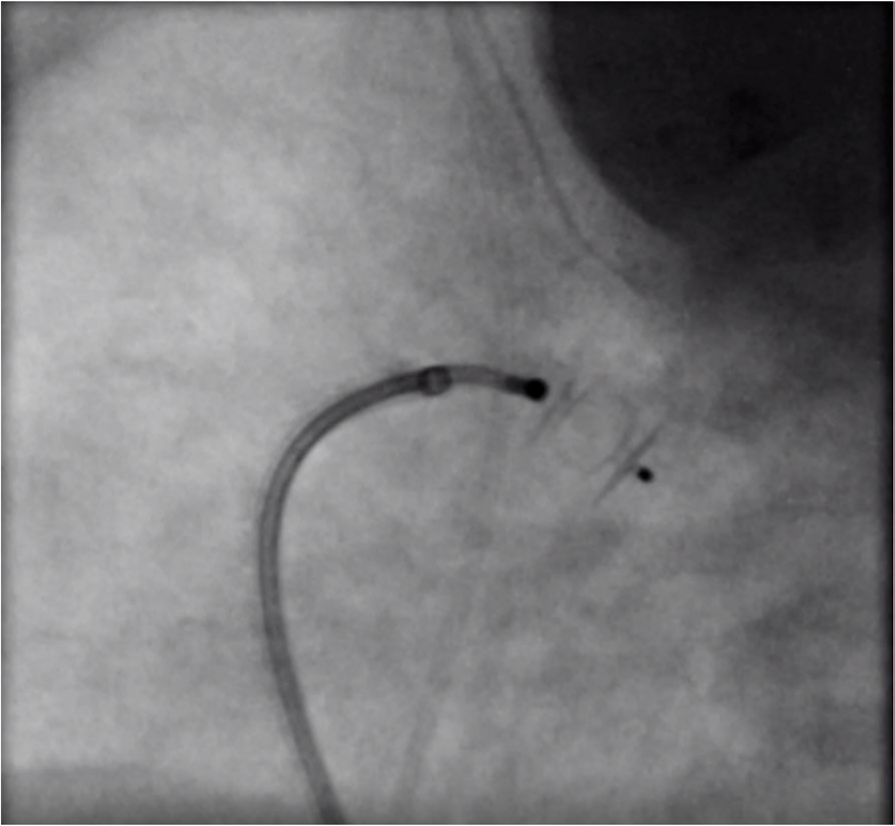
Echocardiographic evaluation right after the release of the device
